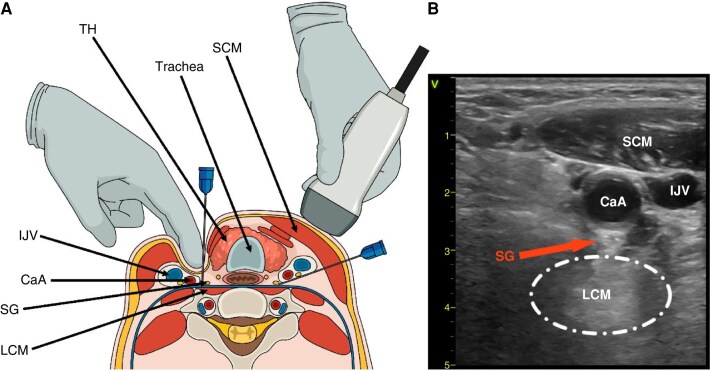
Stellate ganglion block. (*A*) Neck cross-section showing SGB guided by surface landmark technique and palpation (left) and ultrasound (right). (*B*) Transverse sonographic view of the neck at the level of sixth cervical vertebrae (C6). CaA, carotid artery; IJV, internal jugular vein; LCM, longus colli muscle; SCM, sternocleidomastoid muscle; SG, stellate ganglion; SGB, SG blockade; TH, thyroid.
